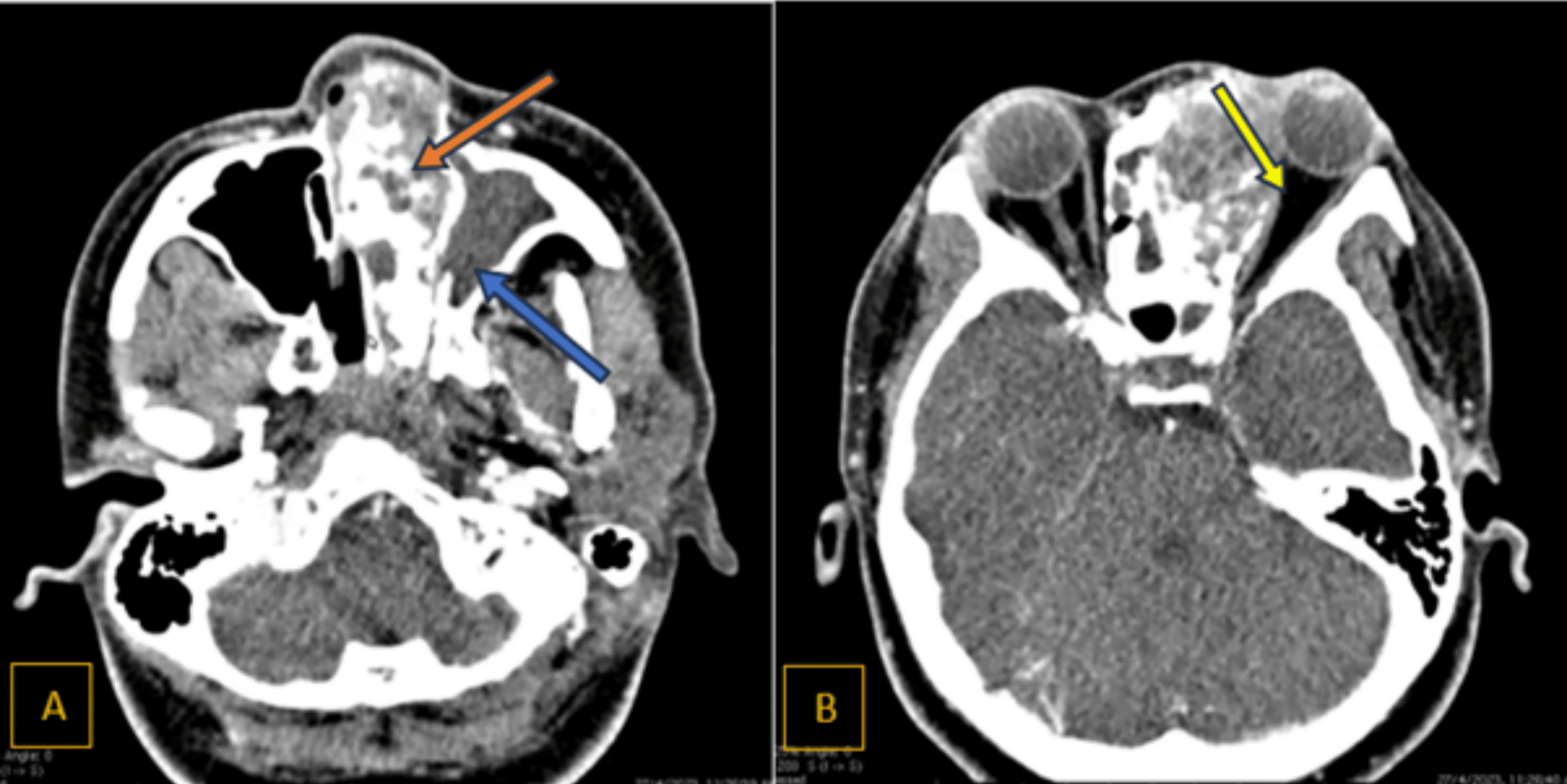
Axial view of CT with contrast (A) Orange arrow: heterogeneous enhancing mass over left nasal space. Blue arrow: erosion of the medial wall of the maxillary sinus with extension into the left maxillary sinus. (B) Yellow arrow: mass abutting the medial rectus muscle on the left side; however, the muscle is preserved CT: computed tomography
